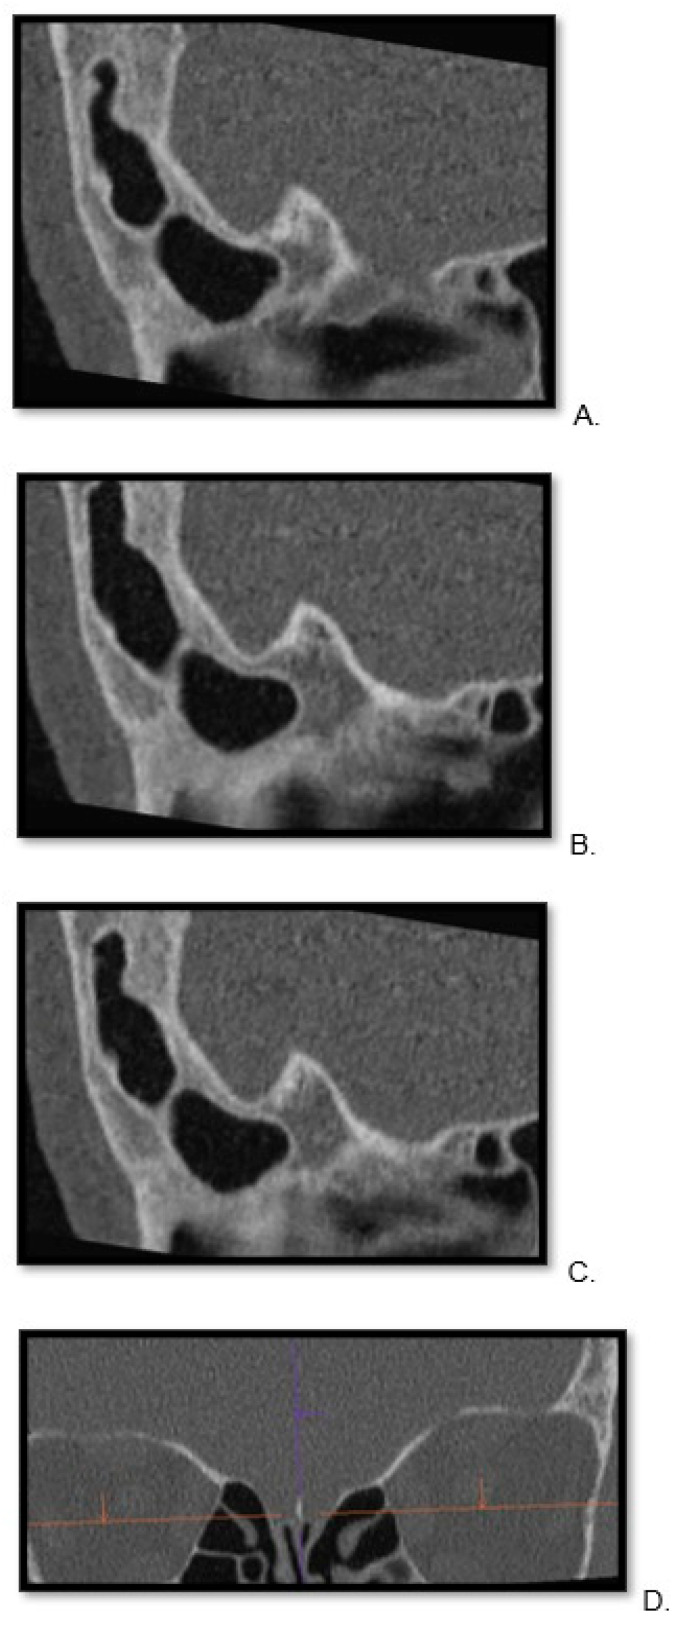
Visualization of cribriform plate in energy-integrated detector computed tomography scan. A lateral visualization of the right to left portions of the cribriform plate in sagittal view (A)—right, (B)—middle, (C)—left side of cribrofirm plate). (D) To facilitate referencing specific layers in sagittal imaging with 0.6 mm, we include a coronal view.
